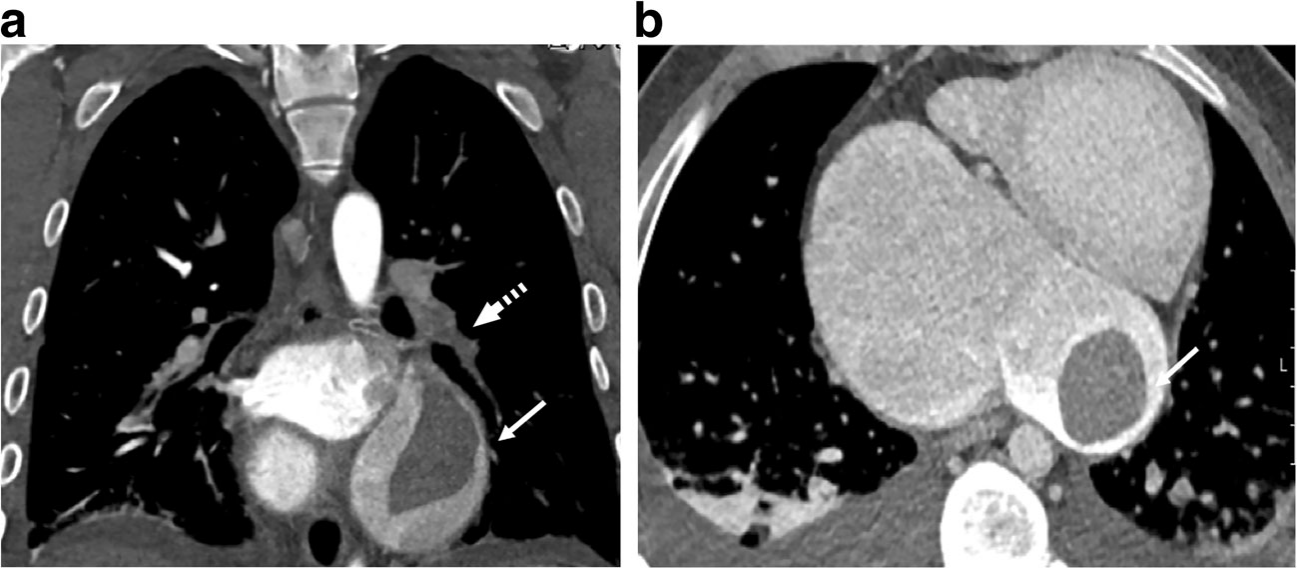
Thromboembolic event in an 18-year-old male Fontan patient with tricuspid and pulmonary atresia. a, b CT angiography. Coronal (a) and axial (b) images reveal a thrombus on the left side of the atrium (*solid arrows*), pulmonary embolus in the left lower branch of the pulmonary artery (*dashed arrow*) and bilateral pleural effusion
